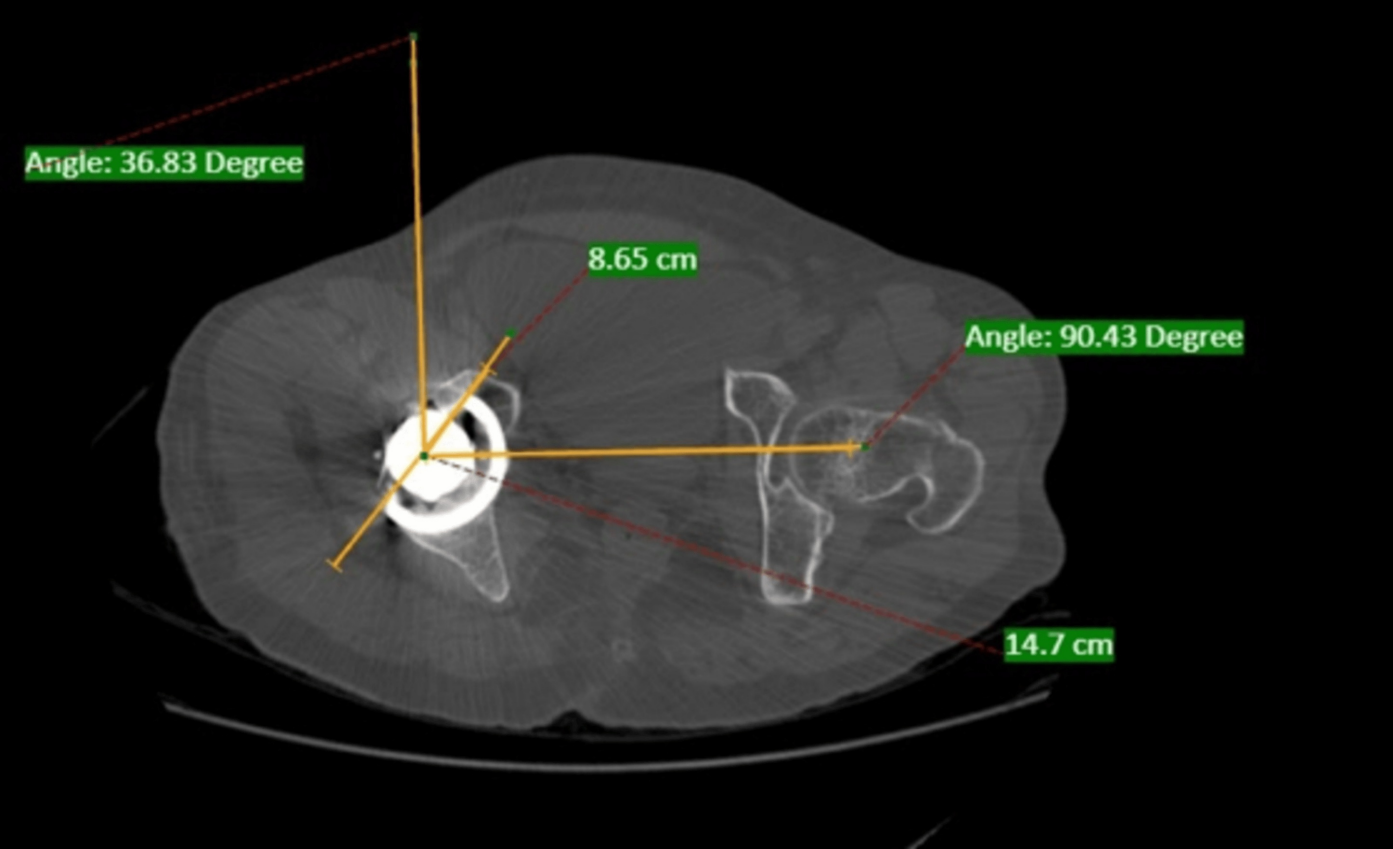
CT scan done in our study
